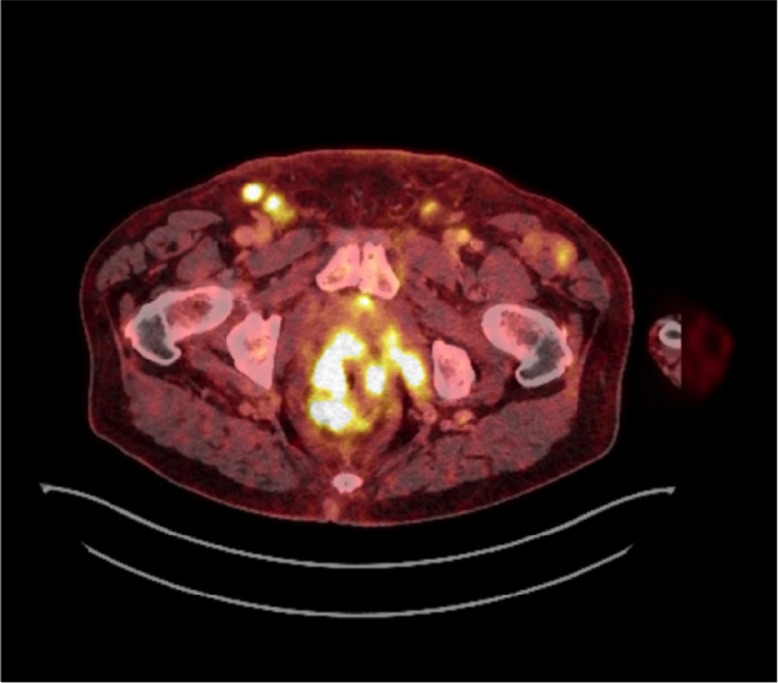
Abdominal PET-CT scan showing a rectal mass, moderate quantities of ascites, and enlarged lymph nodes in pelvis and groin.
